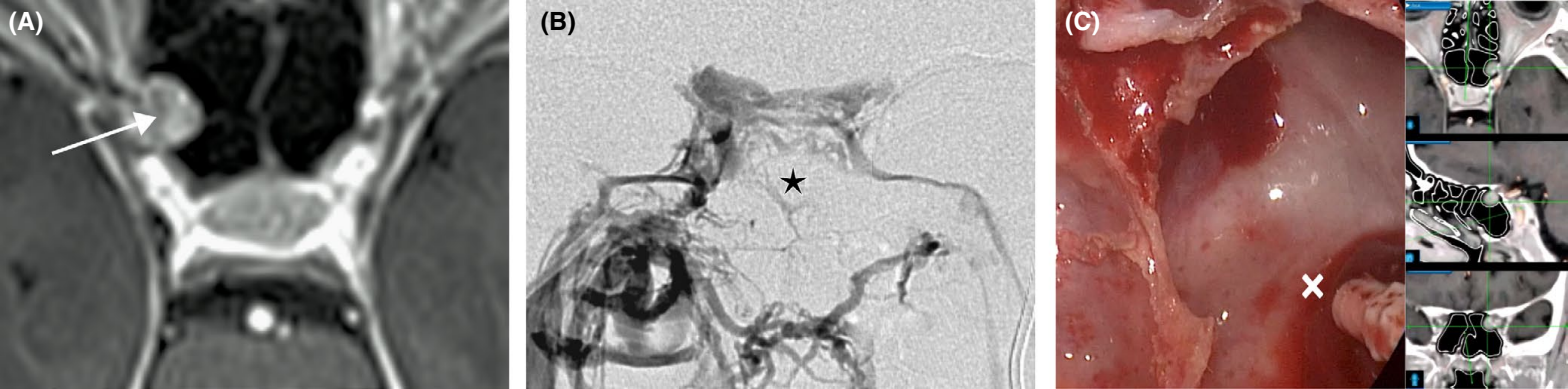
Ectopic corticotroph adenoma in the right sphenoid sinus. A, Axial, contrast‐enhanced MRI of the sphenoid sinus, and sellar region, showing a normal pituitary gland without evidence of any adenoma and a contrast‐enhanced polypoid structure on the right sphenoid sinus wall (arrow). B, Inferior petrosus sinus sampling (contrast agent application through a microcatheter in the right inferior petrosus sinus) showing a vein coming from the polypoid structure in the right sphenoid sinus (asterisk). C, Intraoperative imaging at the time of removal of the polypoid structure in the sphenoid sinus (cross). At the right part of the picture, additional multimodality imaging is provided
